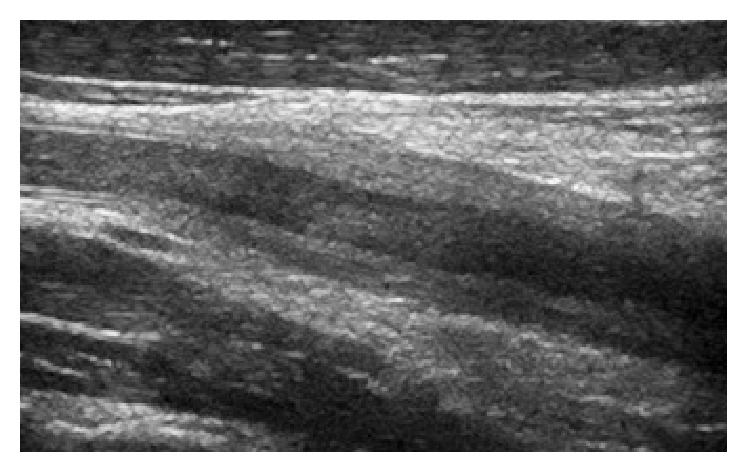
A circumferential thickening of the common carotid artery wall was detected on Doppler ultrasonography, while the vessel wall of the internal carotid artery was normal.
